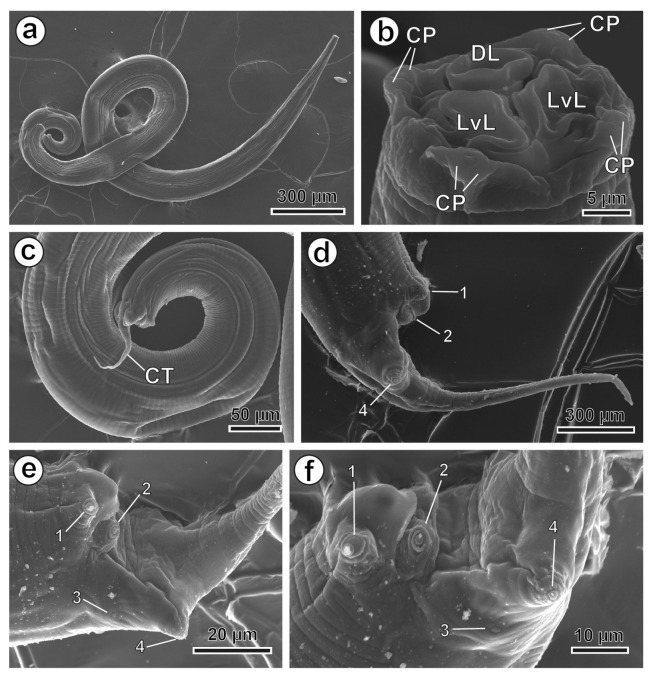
Hilgertia hilgerti* male, SEM. (a) Entire worm. (b) Cephalic extremity showing the three lips, two latero-ventral and one dorsal. (c) Lateral view of the posterior end. (d–f) Different orientations of the cloacal area showing the four pairs of papillae. (CP) cephalic papillae; (CT) caudal tip; (DL) dorsal lip; (LvL) latero-ventral lip; (1 to 4) pairs of caudal papillae.
